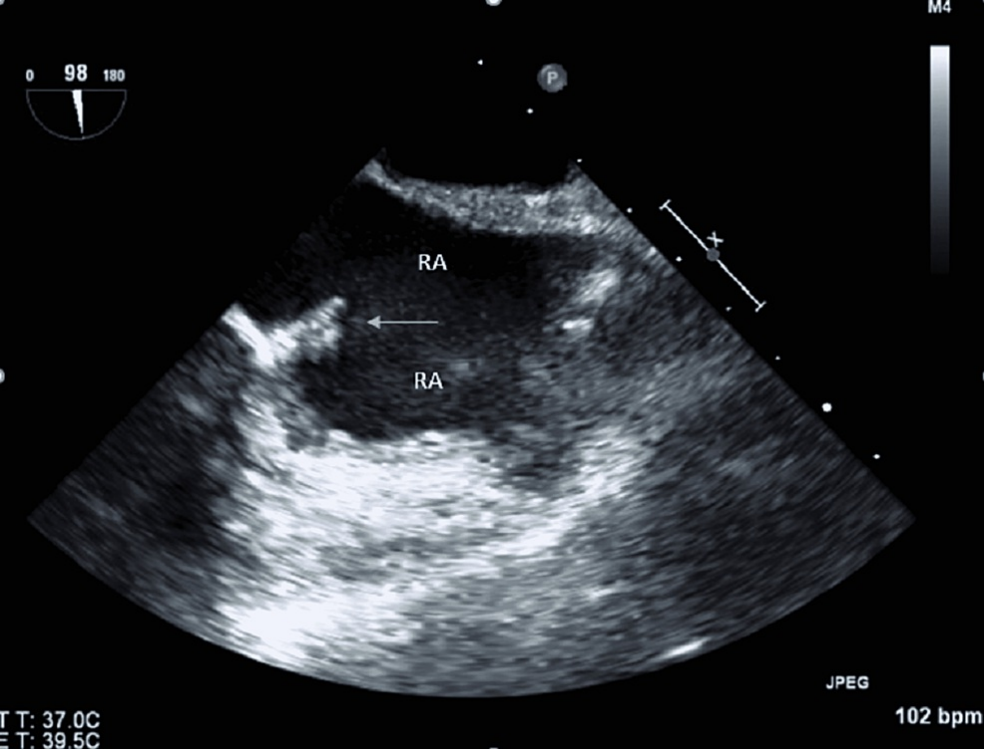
Trans-esophageal echocardiogram (TEE) showing a decrease in the size of the biofilm in the right atrium after the antibiotic therapy. RA, right atrium
